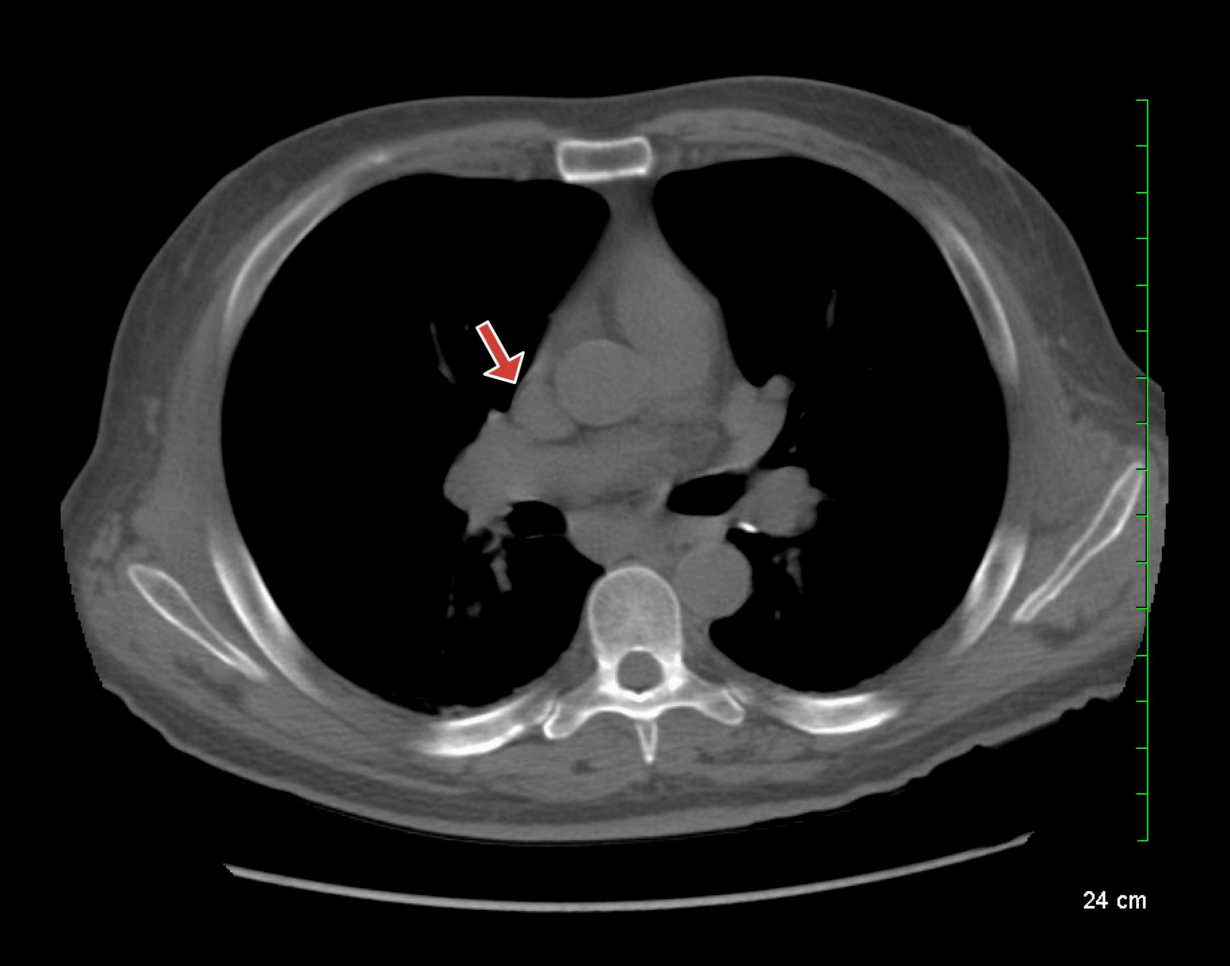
A CT scan of the chest without contrast; mediastinal lymphadenopathy is noted (red arrow).
